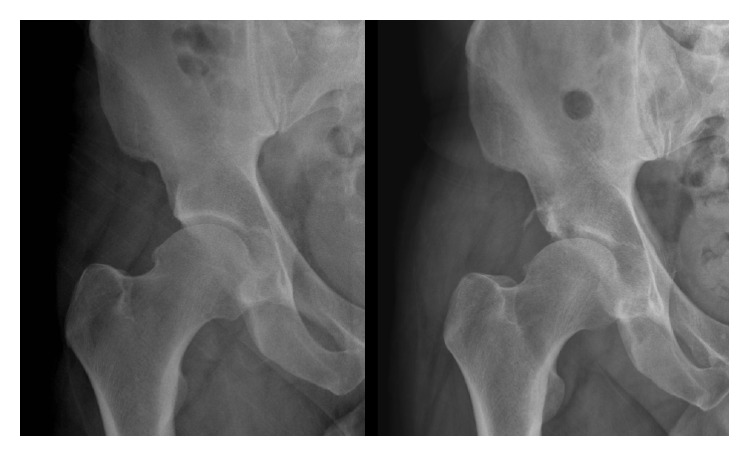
Plain radiograph showing progressive osteolysis of the superior right acetabular rim consistent with chronic infection.
